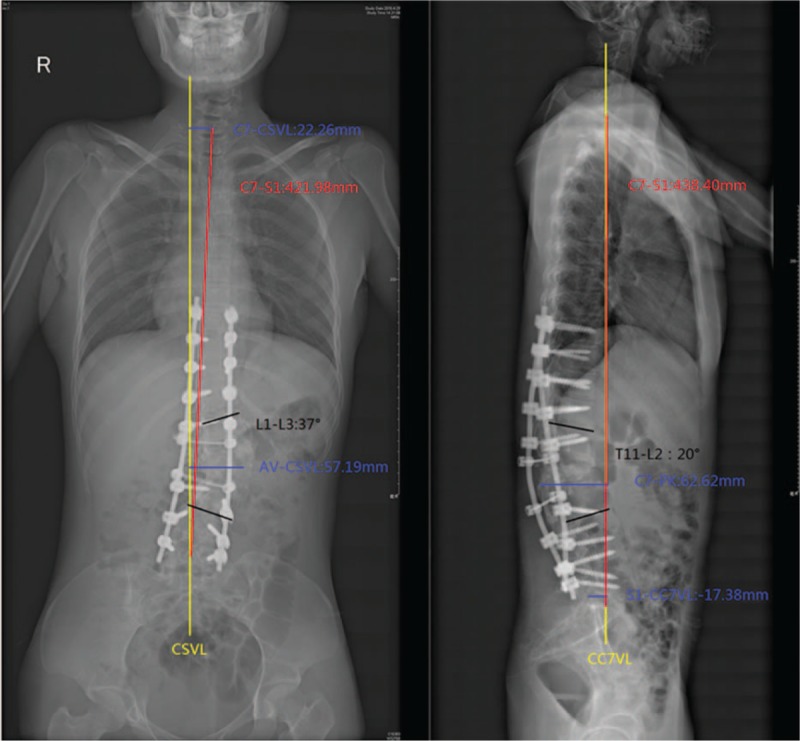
Lateral and anteroposterior X-ray, postrevision surgery.
